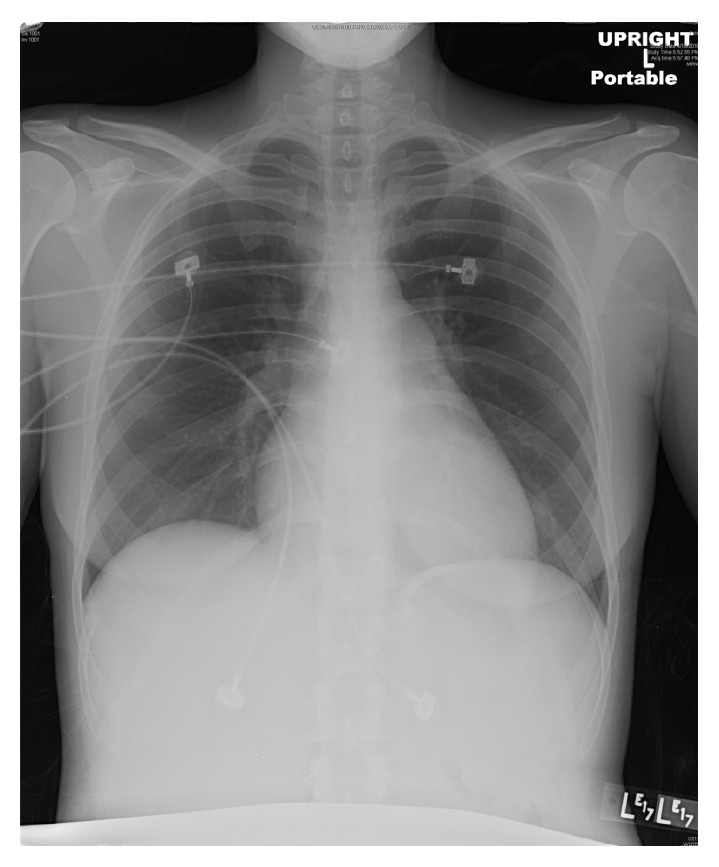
Initial chest X-ray showed no acute findings.
